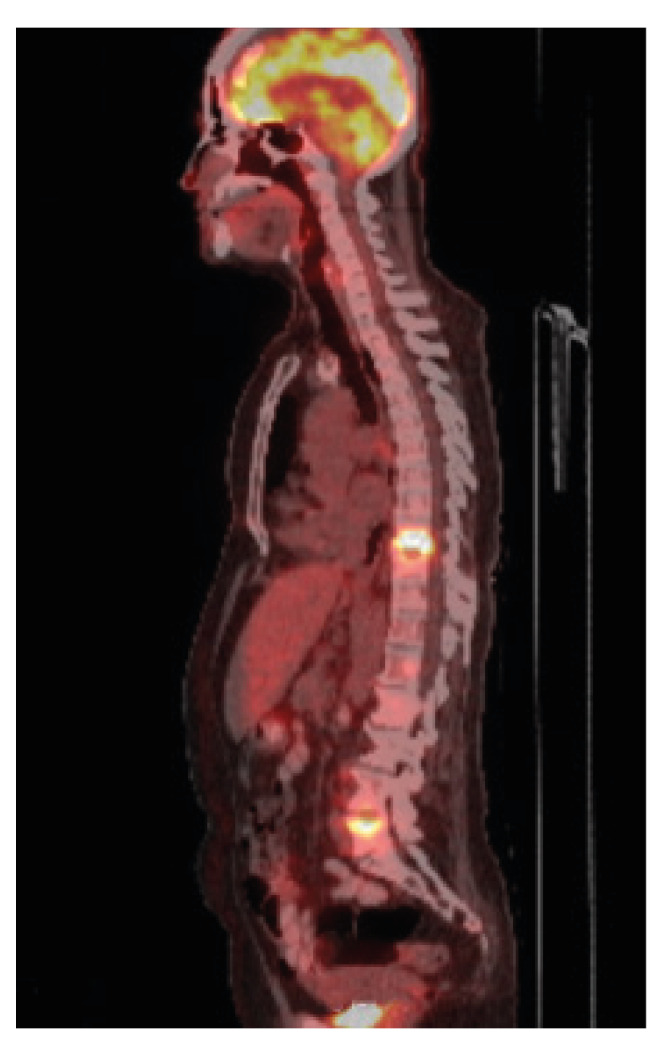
Patient two. Fluoro-deoxy-glucose positron emission tomography (FDG-PET). Increased FDG uptake was observed at T_9_, T_10_, and L_5_ with a maximum standardized uptake value of 20. This finding represents an inflammatory process in which cells consume high levels of glucose.
